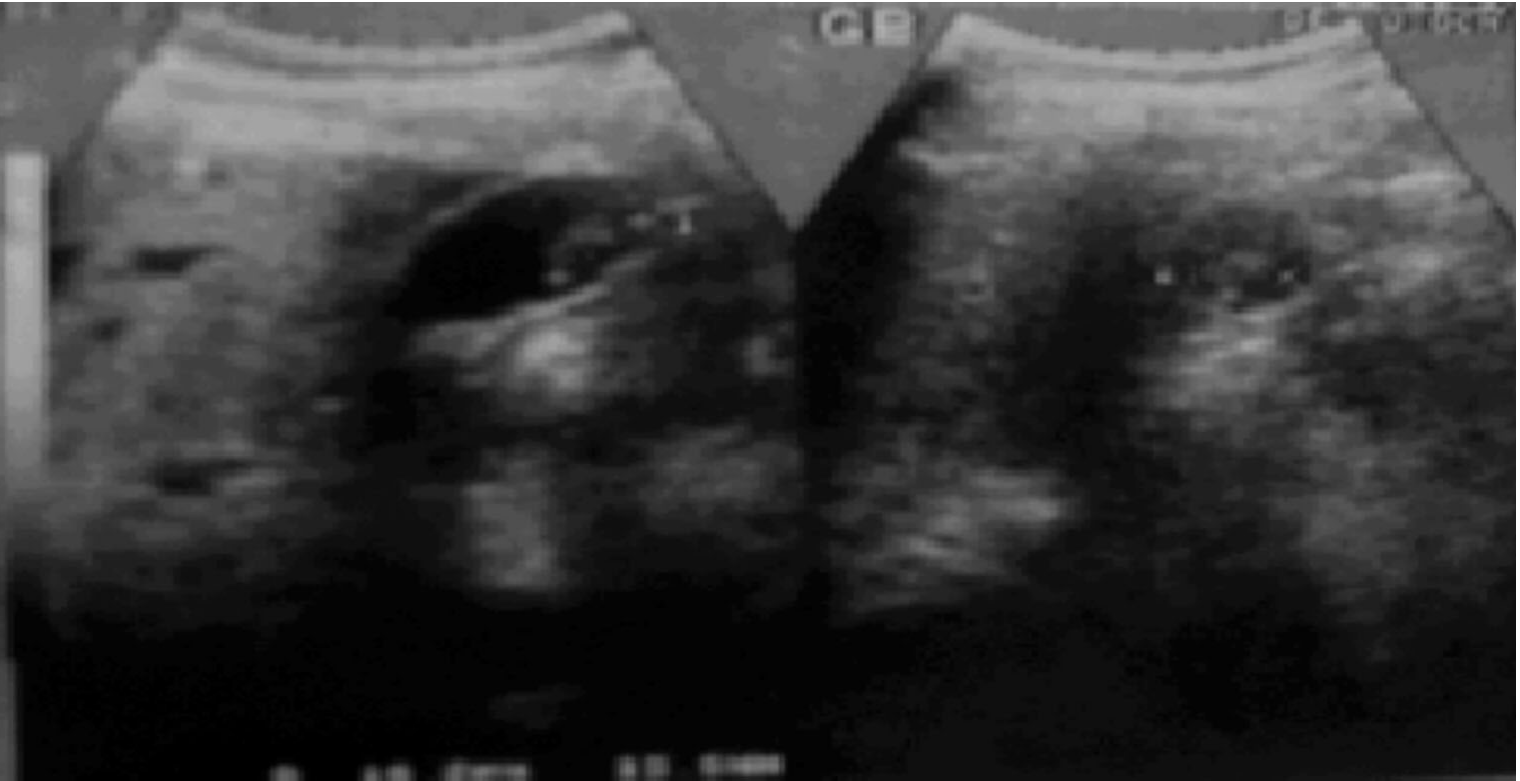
Ultrasonography showing solitary metachronous metastasis to gall bladder.
